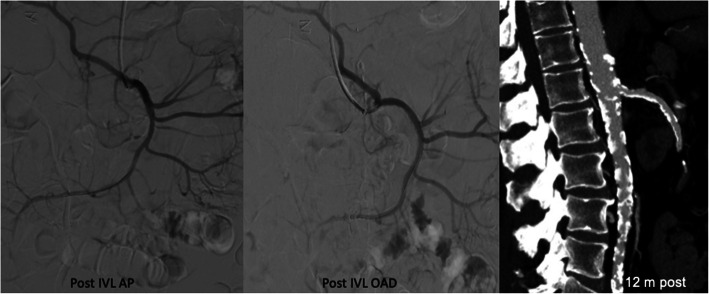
Control arteriography post-treatment with IVL, and MPR of CTA 12 months post-treatment
